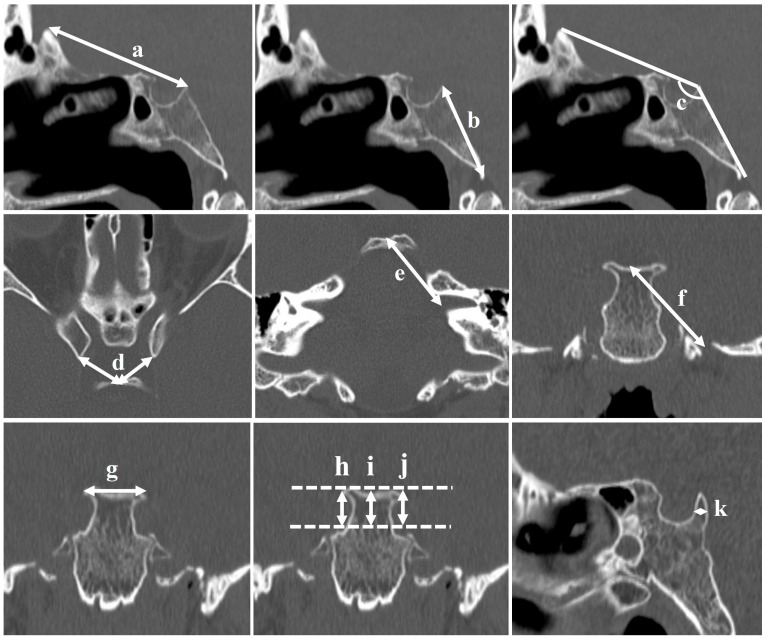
Measured parameters on standardized multiplanar reformatted CT images obtained using calibrated PACS measurement tools: a: DS-CG; b: DS-FM; c: CG-DS-FM; d: DS-ACP; e: DS-IAM; f: DS-FO; g: rPCP-lPCP; h: RLM-H; i: ML-H; j: LLM-H; k: ML-T.
